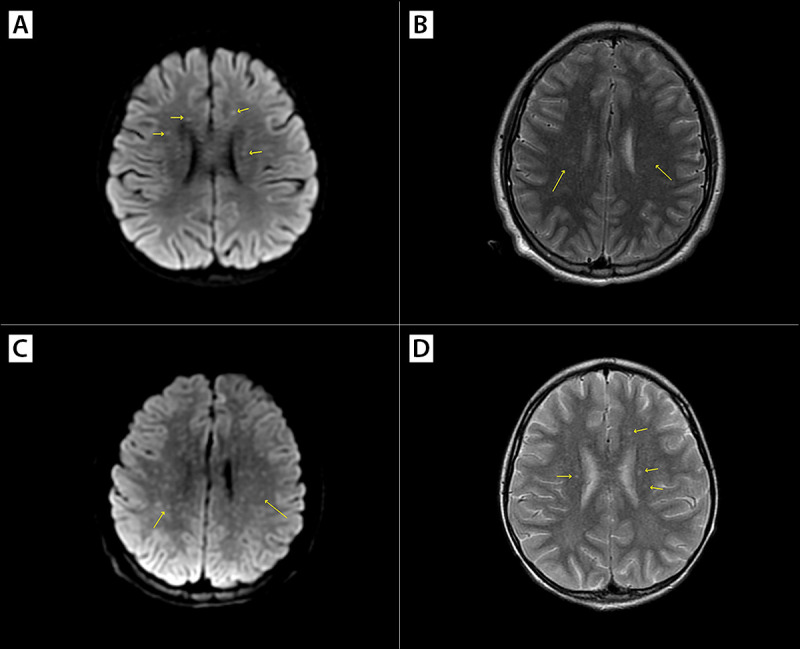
Magnetic resonance imaging findings*^,^^†^ for cases of Rocky Mountain spotted fever in two residents of Tecate, Mexico (a child aged 3 years [A and B] and an adolescent aged 13 years [C and D]) with small foci of signal abnormality in the white matter indicated (“starry sky” appearance)^§^ — California, August 2023 * Axial diffusion-weighted imaging, panels A and C. ^†^ Axial T2-weighted imaging, panels B and D. ^§^ Multiple punctate foci of reduced diffusivity and edema, suggesting white matter ischemic foci associated with vasculitis.
